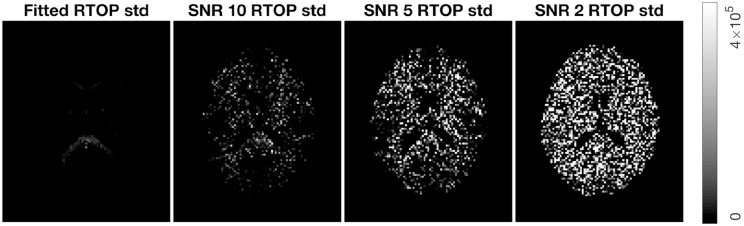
Standard deviation of RTOP for MAP-MRI fitted signal of subjects MGH-1010, slice 45, using 500 bootstrap samples. Gaussian noise of SNR = 10, 5, and 2 were added.
